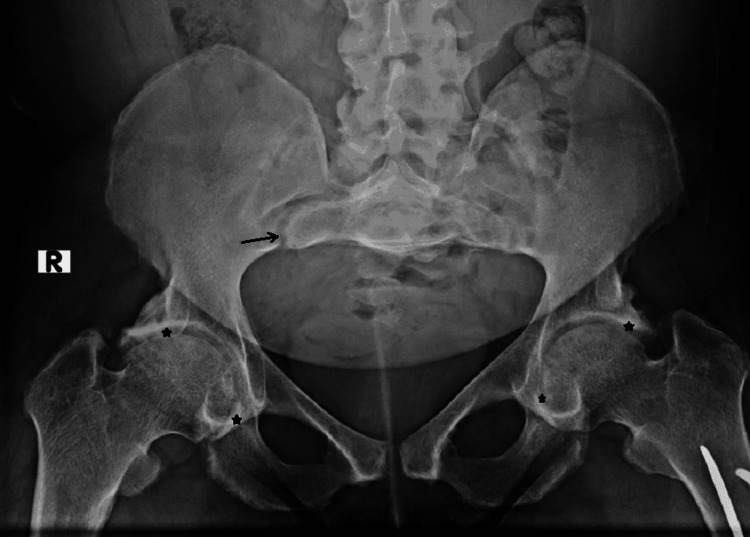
Plain film radiography of the pelvis showed moderate bilateral hip osteoarthritis (stars) and chronic erosive changes in the sacroiliac joints (arrow).
